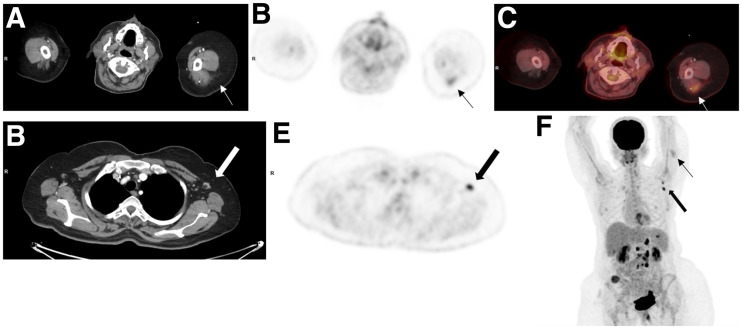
A 52-y-old woman with gastrointestinal tumor was referred for routine ^18^F-FDG PET/CT follow-up study. Study was performed 2 d after second COVID-19 vaccination. Selected transaxial CT (A and D) and PET (B and E) slices at level of posterior arm uptake and axillary LNs, fused image (C) at level of posterior arm uptake, and maximum-intensity projection (F) demonstrate moderate-intensity uptake in left posterior arm (thin arrows) (SUV_max_, 3.6) and high-grade activity in left axillary nodes measuring 1.0 cm in short axis with benign appearance (thick arrows) (SUV_max_, 7.1). Maximum-intensity projection also shows high-grade ^18^F-FDG activity in retroperitoneal nodes and multiple implants.
